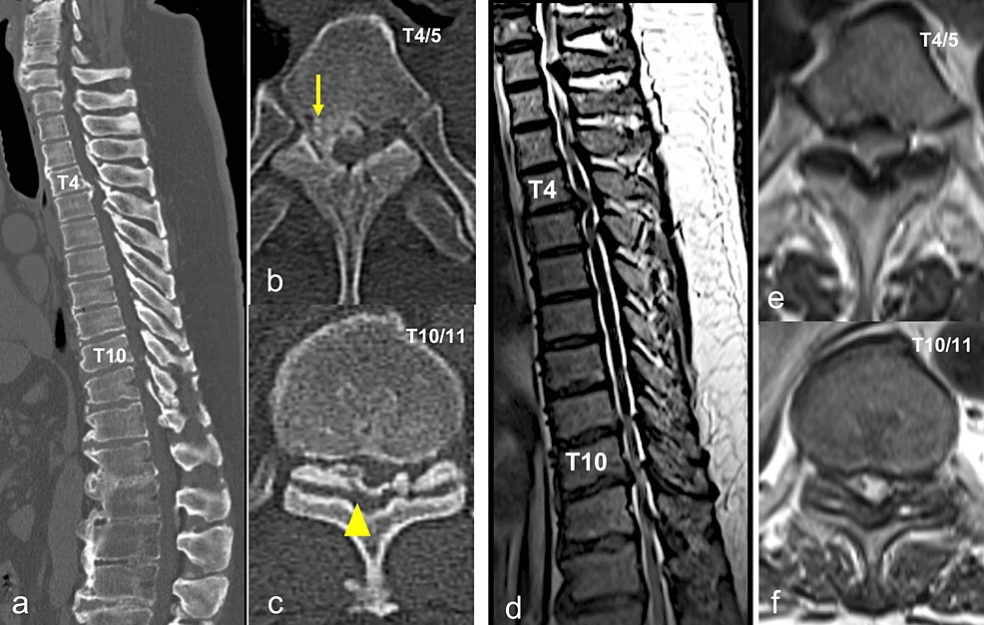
Preoperative images Sagittal reconstruction (a) and axial (b, c) CT show OPLL at T4/5 (yellow arrow) and OLF at T10/11 (yellow arrowhead). Sagittal (d) and axial (e, f) T2-weighed images show significant spinal cord compression at these levels. OPLL: ossification of the posterior longitudinal ligament, OLF: ossification of the ligamentum flavum
